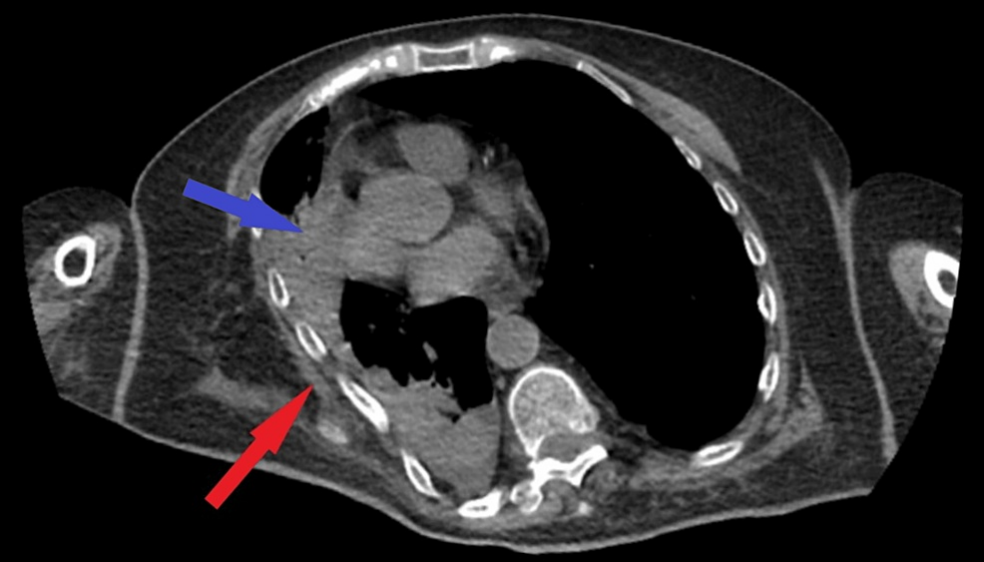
Postoperative pulmonary CT scan After 2 days postoperatively, collapse of the lungs was observed on CT scan consisting of anteriorization of rib arcs (red arrow) and atelectasis lesions (blue arrow)
